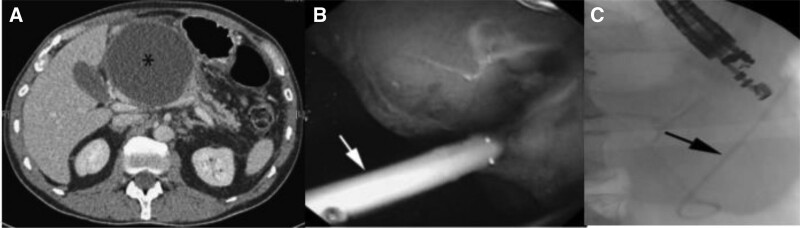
Endoscopic ultrasound in pancreatitis. (A) Computerized tomography image showing pancreatic pseudocyst (asterisk), (B) endoscopic image showing pseudocystogastrostomy plastic stent in situ (white arrow), and (C) fluoroscopic image confirming placement of pseudocytogastrotomy stent (black arrow).
